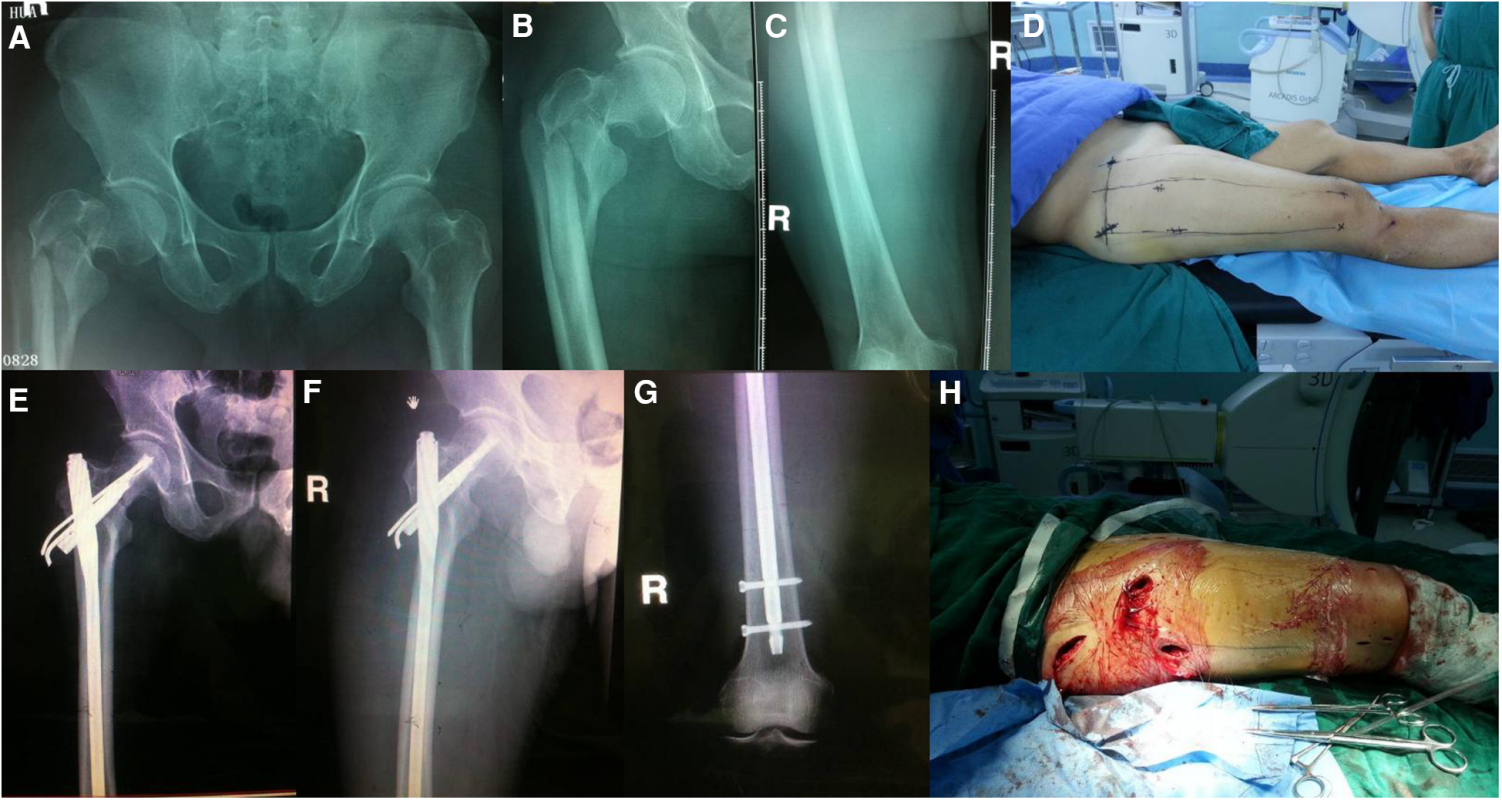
Preoperative anterolateral x-rays of the patient's hip joint (A–C) preoperative localization of skin incision was performed by the “3-2-1” body surface localization method (D) postoperative anterolateral x-rays of the patient's hip joint and left lower extremity showed good fracture reduction (E–G) the postoperative incision was consistent with the preoperative planning, achieving a minimally invasive “3-2-1" (H).
